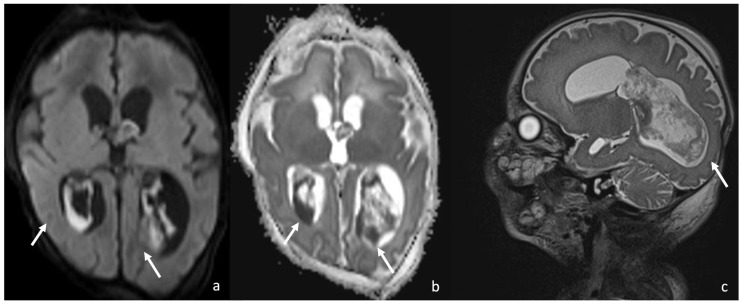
A 10-day-old boy with *Serratia Meningitis,* presenting with plexitis. Axial DWI (a) and axial ADC (b) show infection of the choroid plexus characterized by diffusion restriction (arrows in (a,b)), and sagittal T2 SPACE shows engorgement and infection of the choroid plexus (arrow in (c)).
